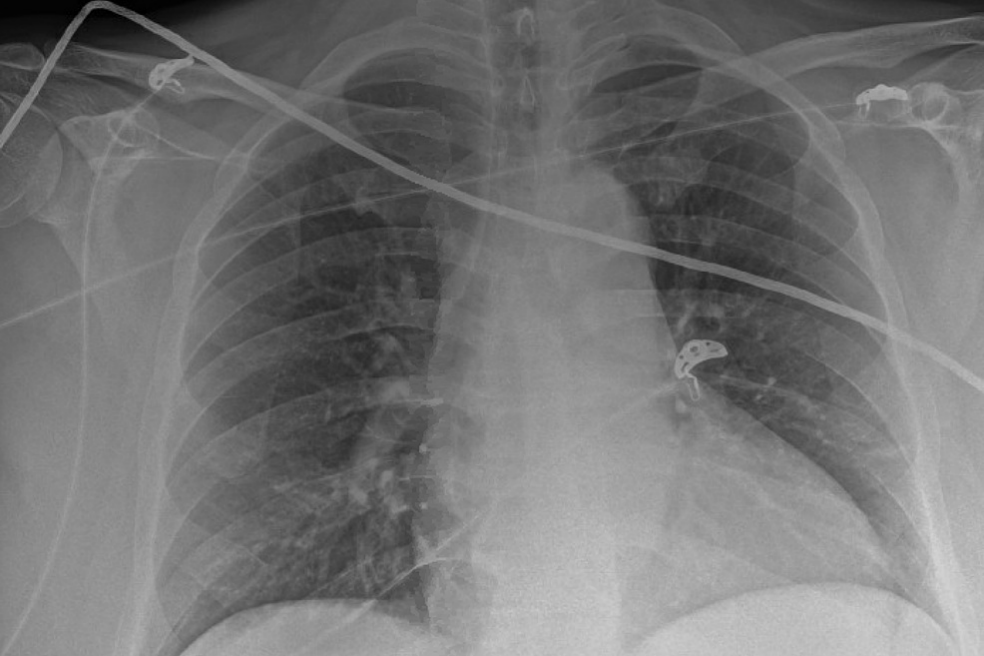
Chest X-ray showing increased bilateral interstitial marking
